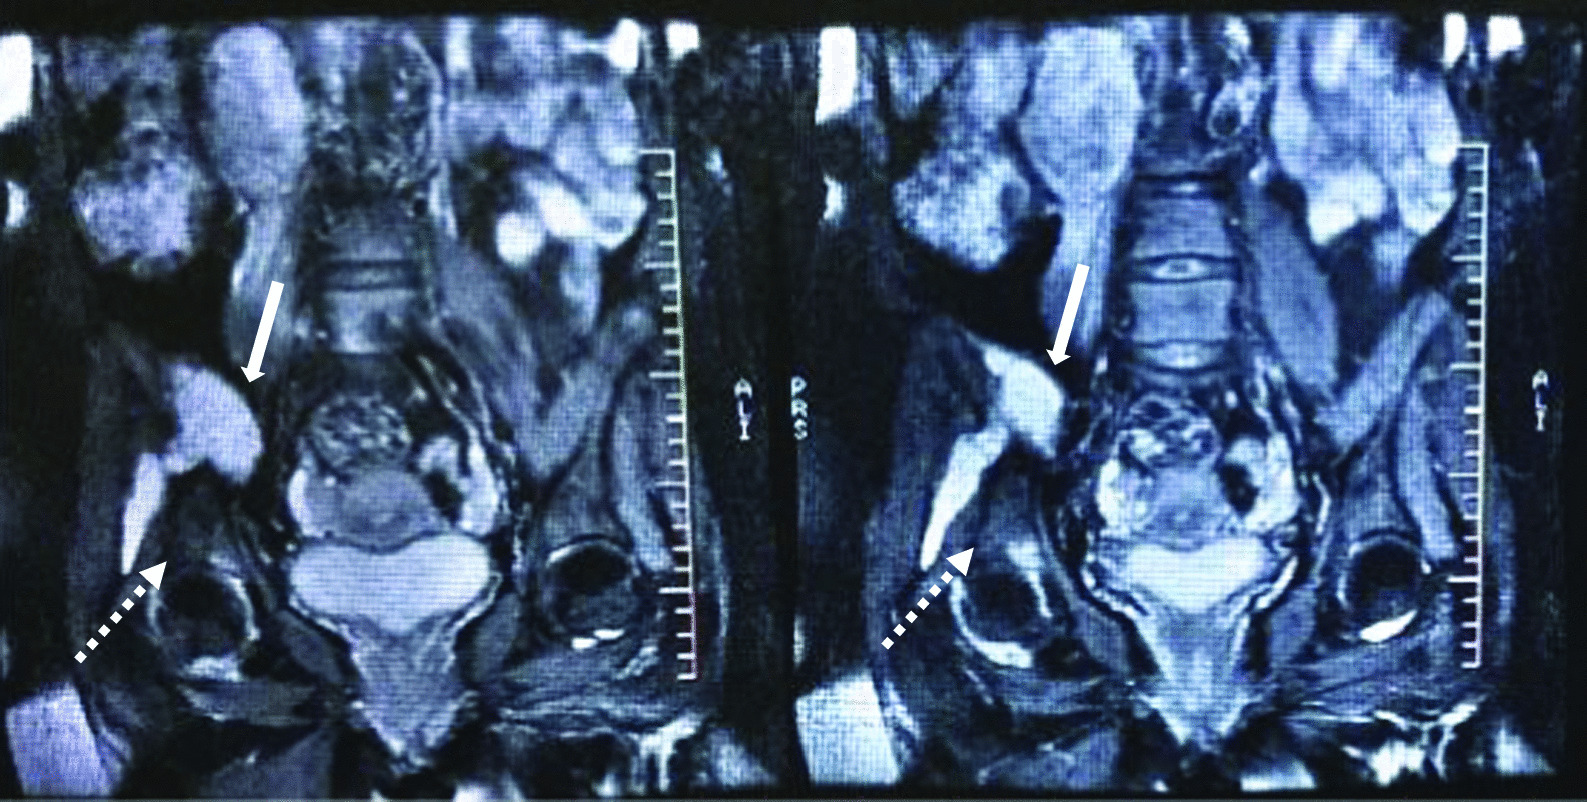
T2 abdominal and pelvic MRI showing a cyst above the right iliac bone (white arrows) extending through it, necrosis of the ilium wing, and joint subluxation between the right head of the femur and the acetabulum due to osteomyelitis (dashed arrows)
